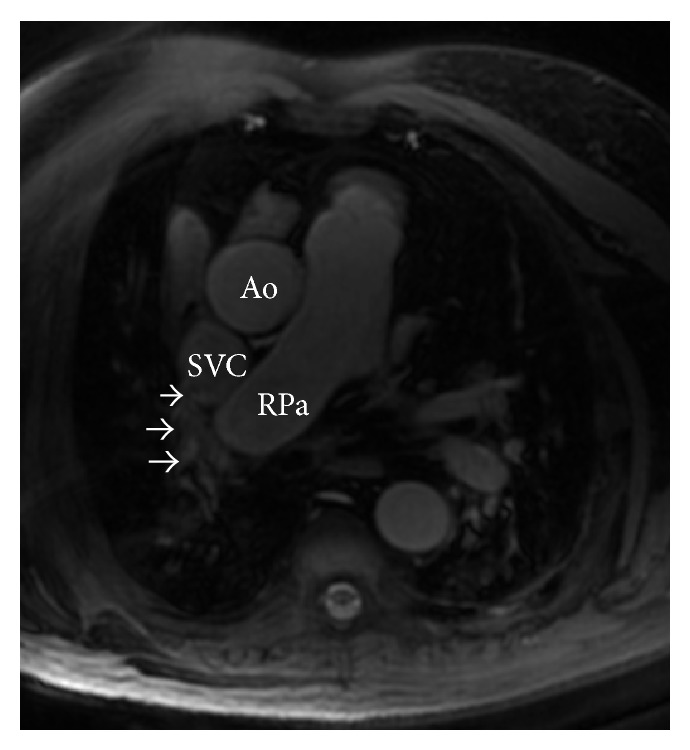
Axial 3-dimensional steady state free precession (SSFP) magnetic resonance image demonstrating anomalous pulmonary venous return of the right superior pulmonary vein into the superior vena cava. Right upper lobe partial anomalous pulmonary venous return (arrows). SVC: superior vena cava; RPa: right pulmonary artery; Ao: aorta.
